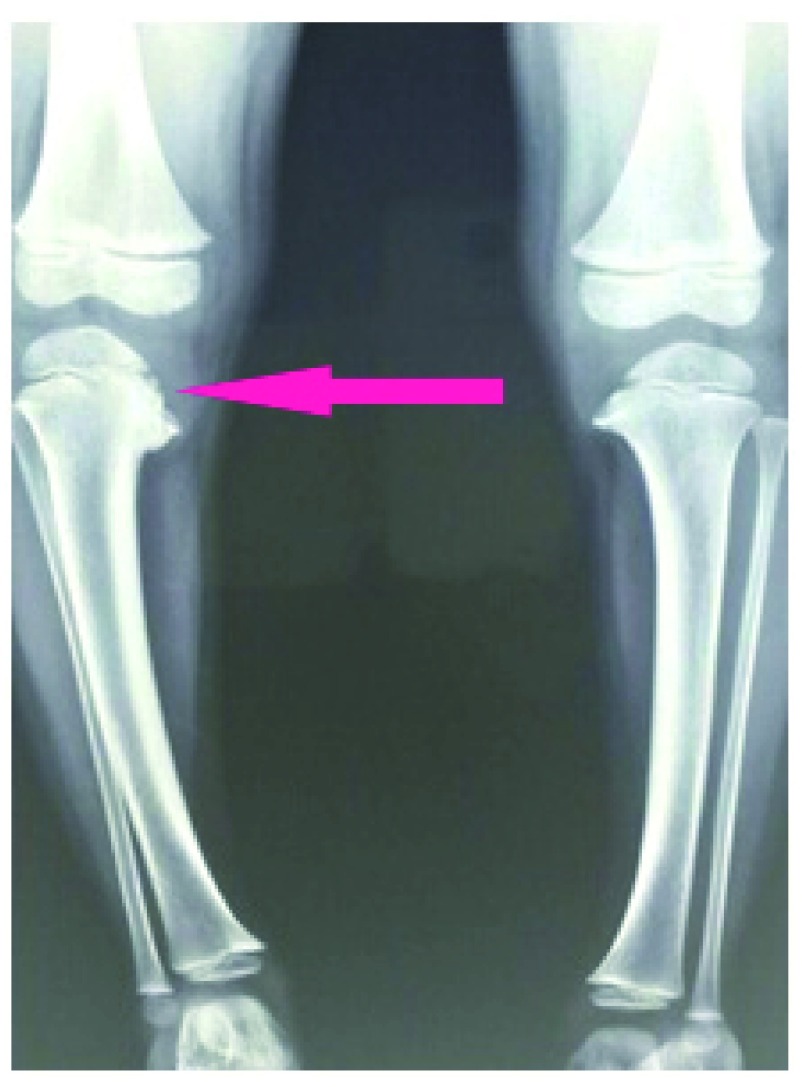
X-ray shows depression of medial tibial plateau with beaking of posteromedial tibial metaphysis.
